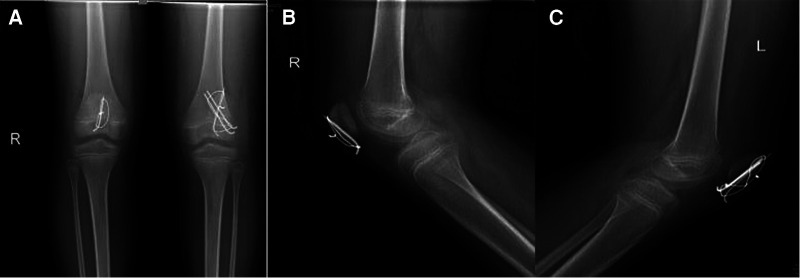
(A) Post-operative AP radiograph of the both knees. (B) Post-operative lateral radiograph of the right knee. (C) Post-operative lateral radiograph of the right knee.
